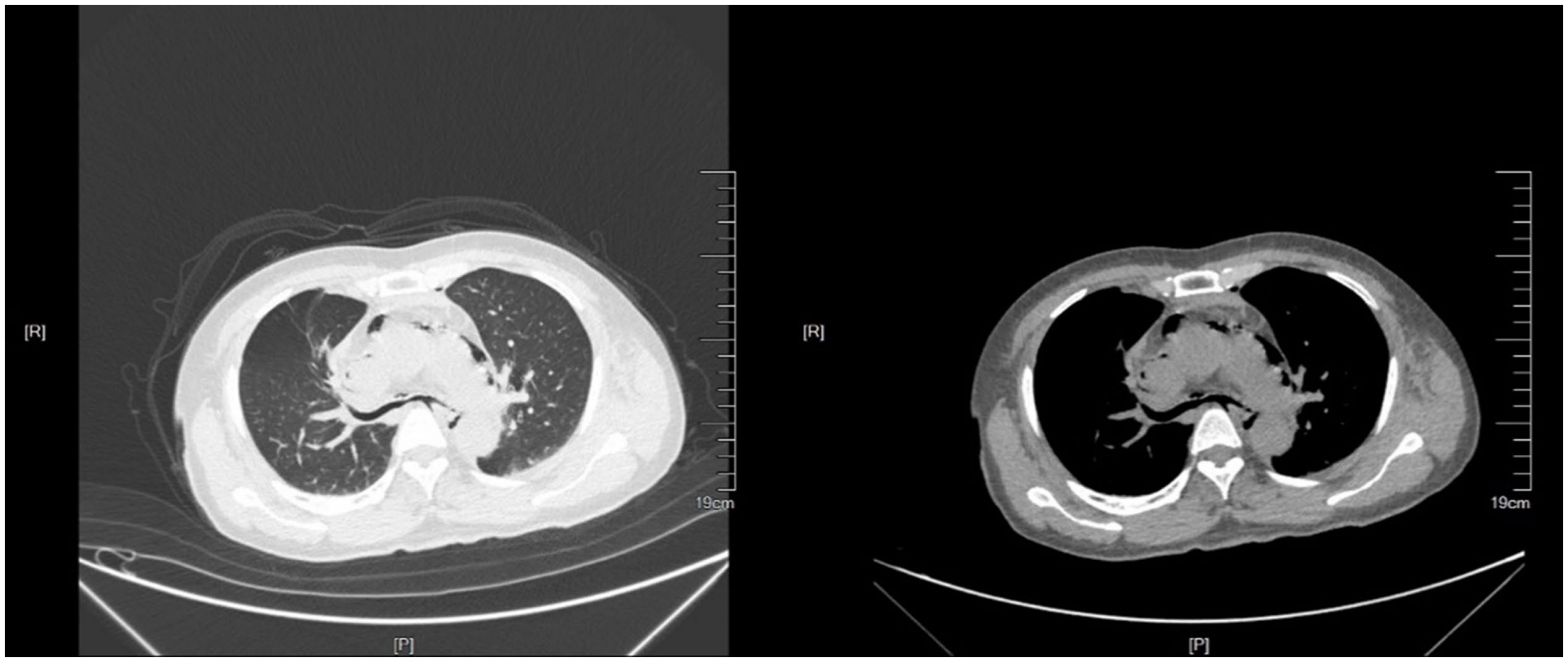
Chest CT at the first visit.
